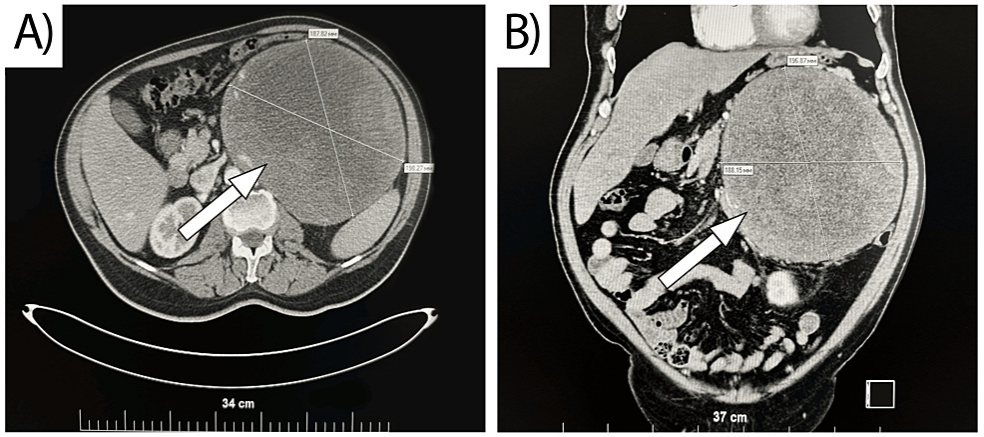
CT of the abdomen with intravenous contrast A) axial plane; B) coronary plane
